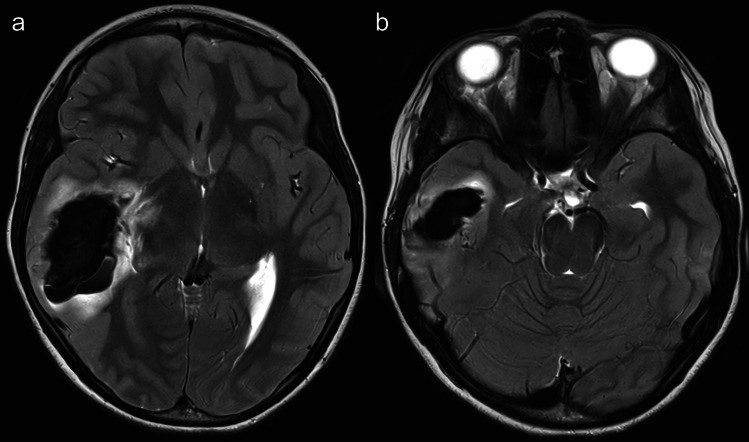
Axial T2-weighted MRI, performed during second presentation at our department, revealed a large intraparenchymal hemorrhage in the right temporal lobe with perilesional edema a at the level of the basal ganglia and internal capsule and b in the temporo-basal region
